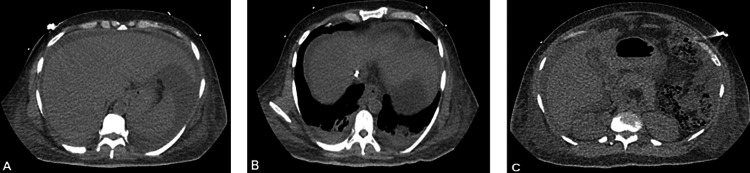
Abdominal computer tomographic angiography (CTA) after liver biopsy findings: (A) Status post-ultrasound-guided core liver biopsy showing the development of new large volume complex attenuating perihepatic ascites fluid tracking along the paracolic gutters into the lower pelvis. This finding may represent blood products. (B) There is an enhancing focal area not seen elsewhere in the liver with a surrounding slight lucency at the periphery of the liver anteriorly suspicious for a site of active extravasation of contrast, likely active bleeding. (C) Diffuse hepatic steatosis with extensive volume complex ascites with centralization of the bowel loops and hematocrit levels in the right paracolic gutter and lower pelvis indicate hemorrhagic ascites or ascites with a hemorrhagic component.
